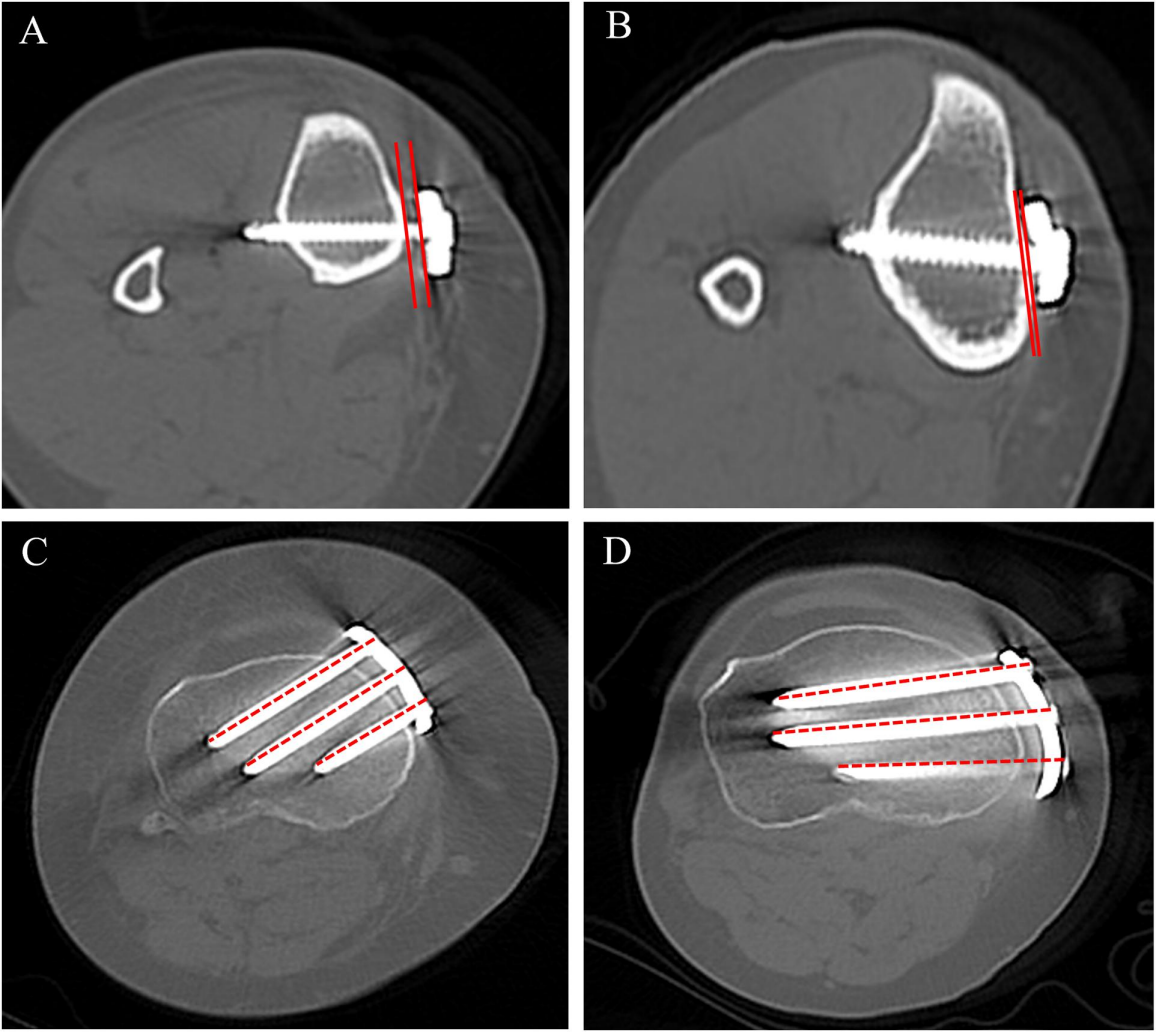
Axial CT measurements of (i) the vertical plate–bone gap (measured immediately inferior to the osteotomy) and (ii) the lengths of screws A, B, and C. (A) T-shaped locking plate-vertical plate–bone gap; (B) novel locking plate-vertical plate–bone gap; (C) T-shaped locking plate-lengths of screws A–C; (D) novel locking plate-lengths of screws A–C.
